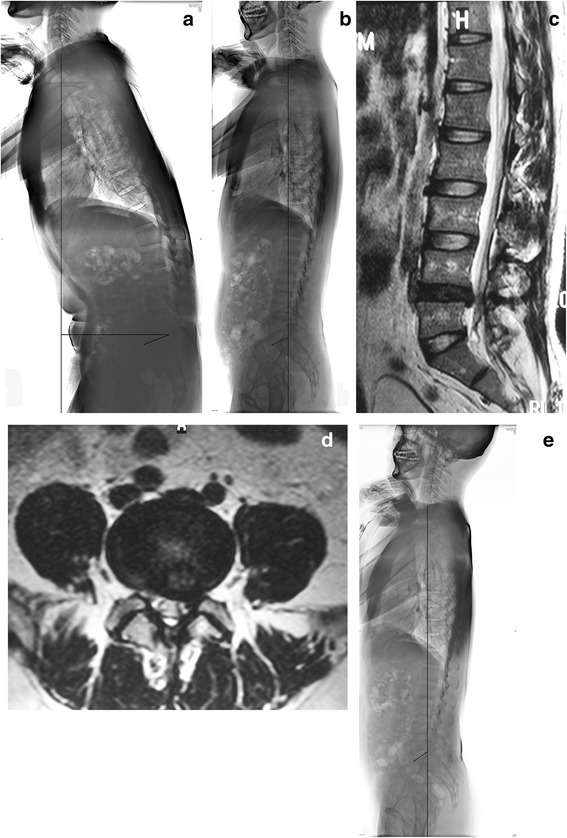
Thirty-three year-old man with L4/5 disc herniation (patient No.2). a Preoperative lateral radiograph showed sagittal imbalance. (SVA 18.3 cm). b Immediately after surgery, radiograph showed restored sagittal balance. (SVA −0.5 cm). c, d MRI revealed L4/5 disc herniation with impingement of the left L5 nerve root. e Lateral radiograph taken 2.5 years after surgery showed sagittal balance. (SVA 0.5 cm)
